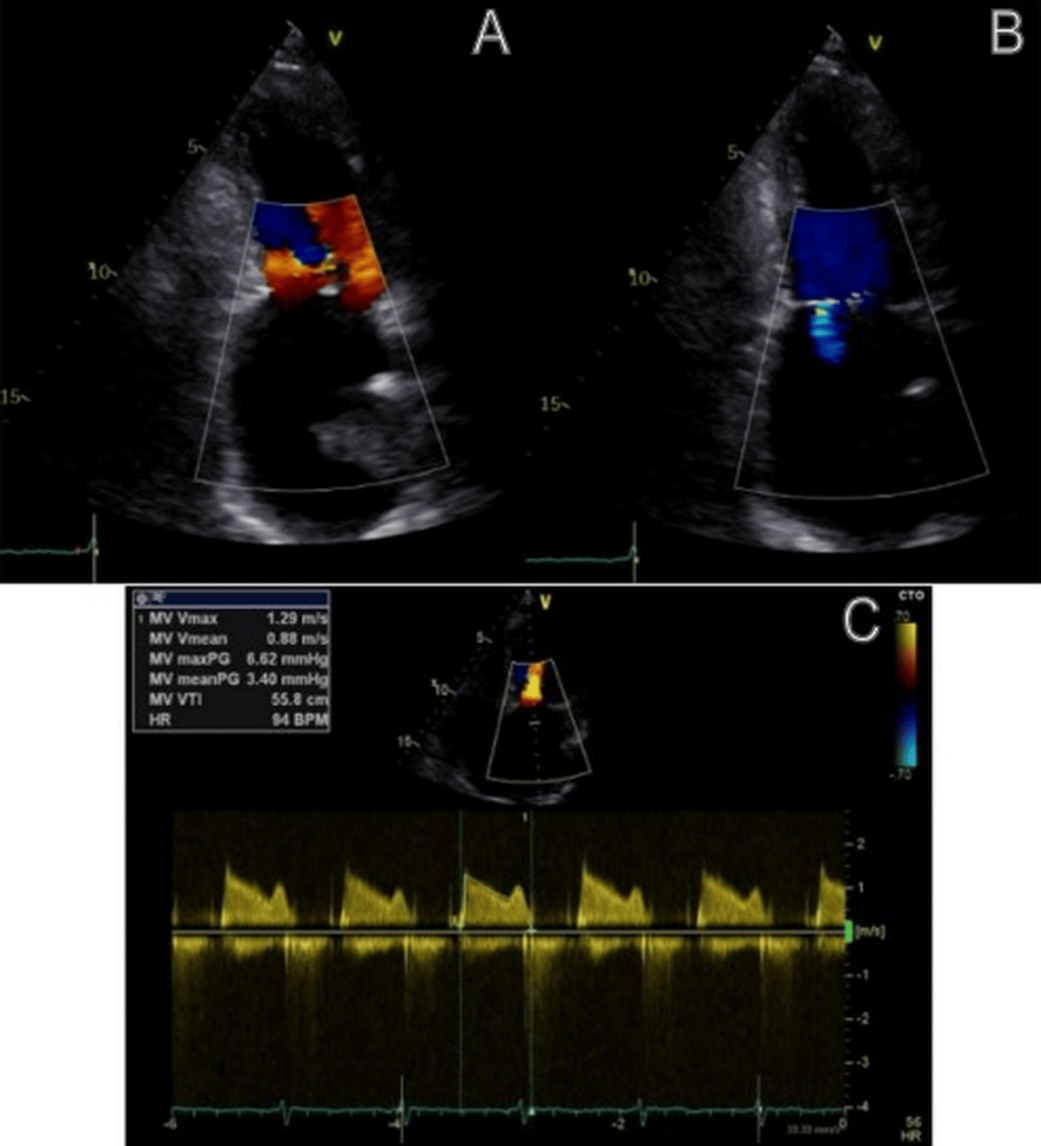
Follow-up imaging findings (A, B) Transthoracic echocardiogram at 1-year follow-up showing trivial mitral regurgitation. (C) Continuous-wave Doppler showing a mean transmitral gradient of 3.4 mmHg
